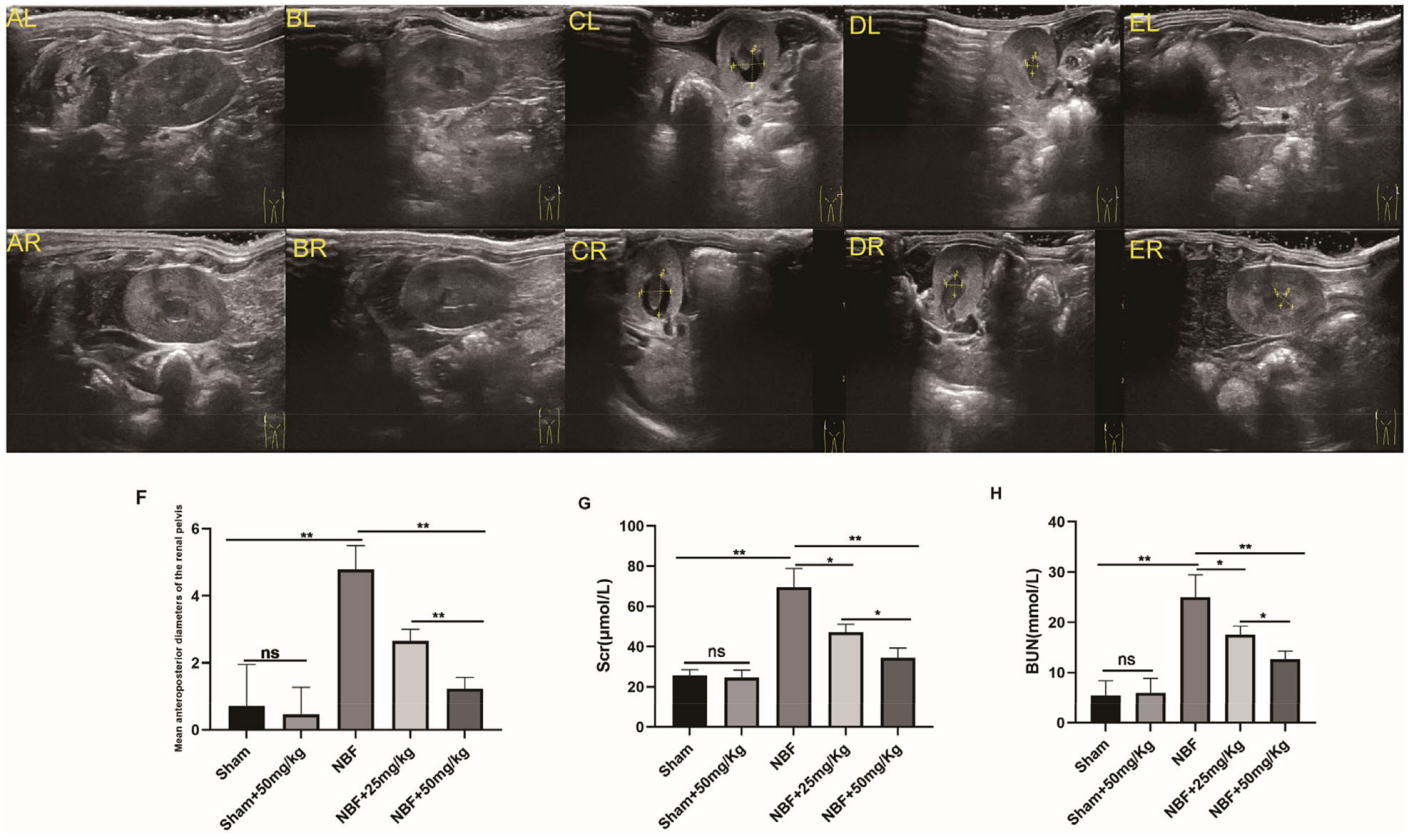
Ultrasound images of different groups show the difference in diameter between the anterior and posterior renal pelvis.ALandAR: left and right kidneys in the sham group; there was no obvious hydronephrosis in the renal pelvis. BLandBR: left and right kidneys in the sham + ABT-263 (50 mg/kg) group; there was no notable hydronephrosis in the renal pelvis. CLandCR: left and right kidneys in the neurogenic bladder fibrosis (NBF) group andhydronephrosis in the renal pelvis. DLandDR: left and right kidneys in the NBF + ABT-263 group (25 mg/kg); compared with the NBF group, hydronephrosis in the renal pelvis was significantly reduced. ELandER: left and right kidneys in the NBF + ABT-263 group (50 mg/kg). Compared with the NBF + ABT-263 group (25 mg/kg), hydronephrosis in the renal pelvis was significantly reduced. The yellow arrow indicates the hydronephrosis area. f: Statistical histograms of the anterior and posterior diameters of the renal pelvis (the anterior and posterior diameters of the left and right kidneys were added together and then averaged); gandh: Statistical histograms of serum creatinine (SC) and blood urea nitrogen (BUN) levels. Data are expressed as the mean ± SEM; ns *p* > 0.5, p* < 0.01, *p* < 0. 001. All experiments were repeated three times.
